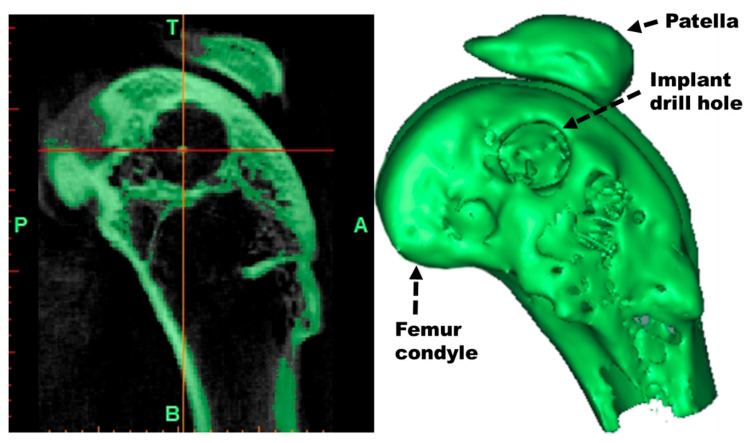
µ-CT image (left) and 3D reconstruction (right).
